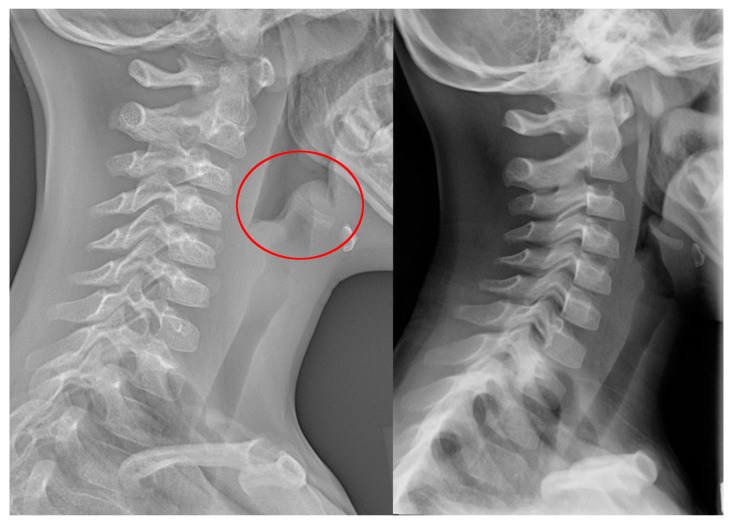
Lateral neck soft tissue radiographs demonstrating epiglottitis and its resolution. (Left): Radiograph on day 0 of admission showing epiglottic thickening with mild pharyngeal soft tissue distension (red ellipse). (Right): Radiograph on day 3 of admission showing resolution of swelling.
